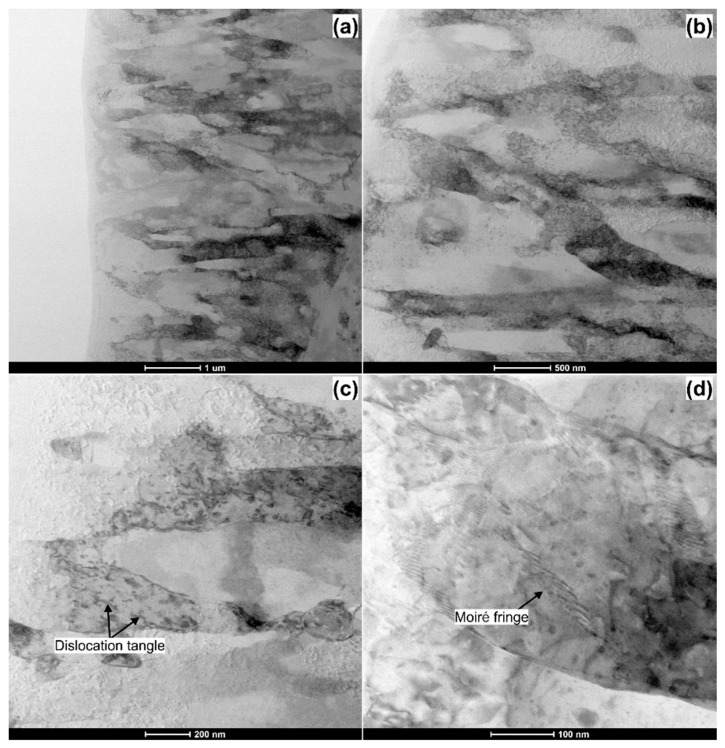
STEM bright-field images of the AlMg3 sample deformed with the action of ultrasonic vibrations. (a) low magnification image of the deformed area; (b) high magnification image of the deformed area; (c) magnified image of the deformed area showing a dislocation tangle; (d) magnified image of the deformed area showing a Moiré fringes.
